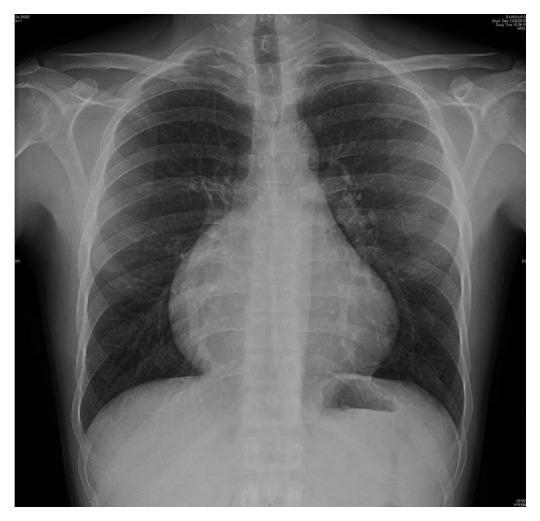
Chest radiograph showing cardiomegaly with right atrial enlargement.
